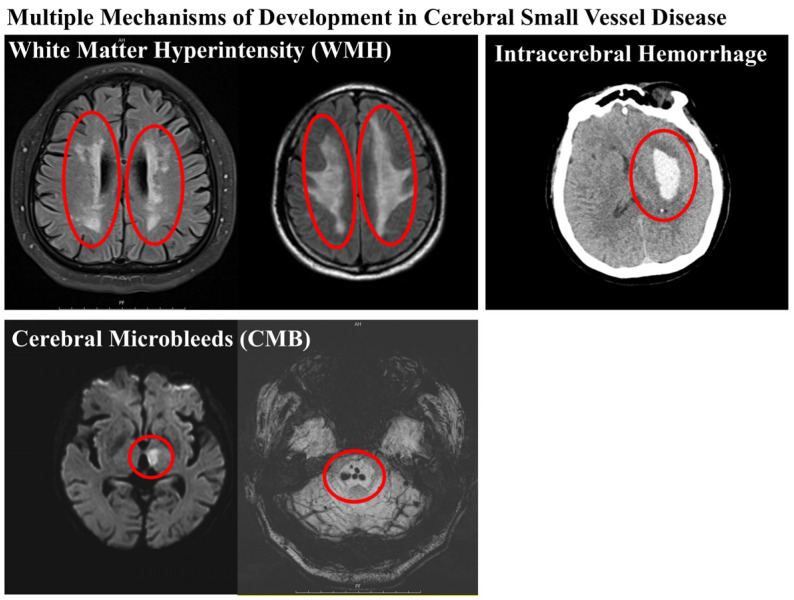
Brain MRI images of patients who have experienced CSVD. WMH, CMB, lacunar infarct, and ICH worsen CSVD due to ischemic or hemorrhagic lesions. The red circles within the brain are cerebral hemorrhage and ischemic conditions identified by WMH, CMB, infarct, and hemorrhage occurrence points, respectively.
